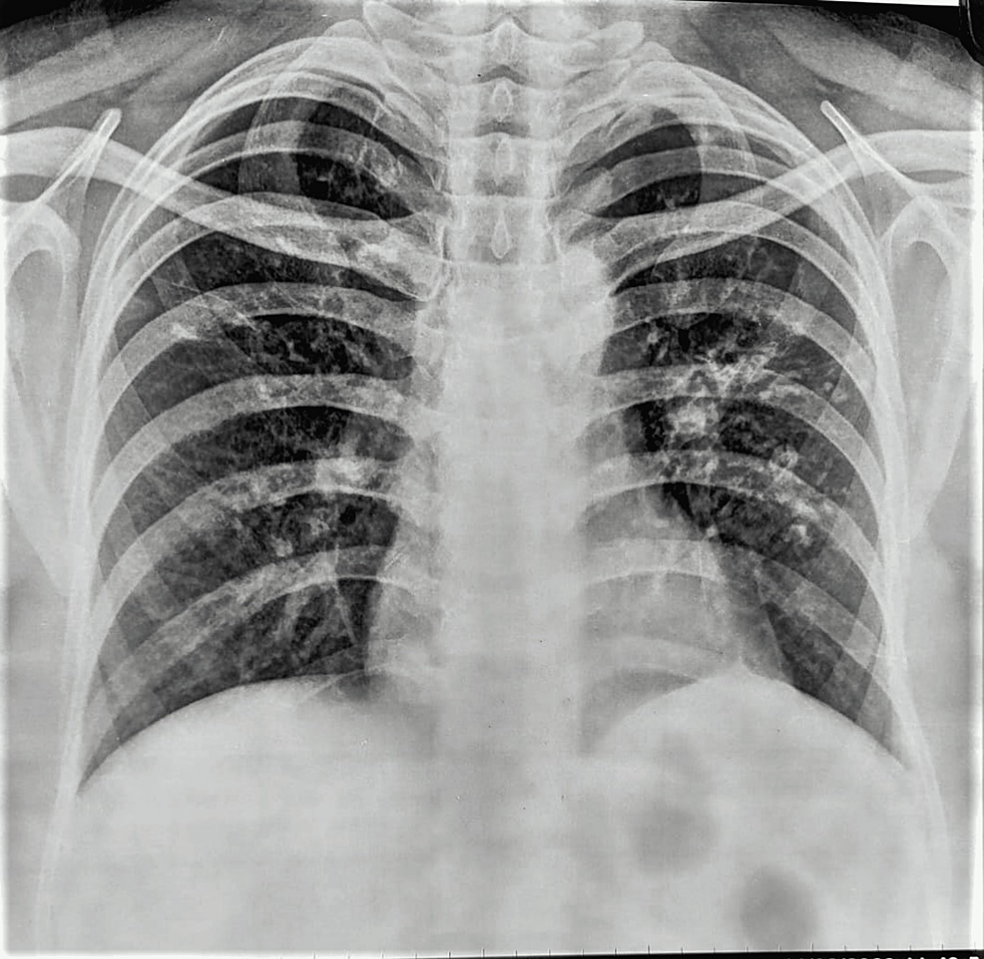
Chest X-ray X-ray shows bilateral calcified and fibrotic lesions involving right upper, middle, and lower zones and in left upper and middle zones with evidence of hyperinflation, bronchial wall thickening in right lower zone.
